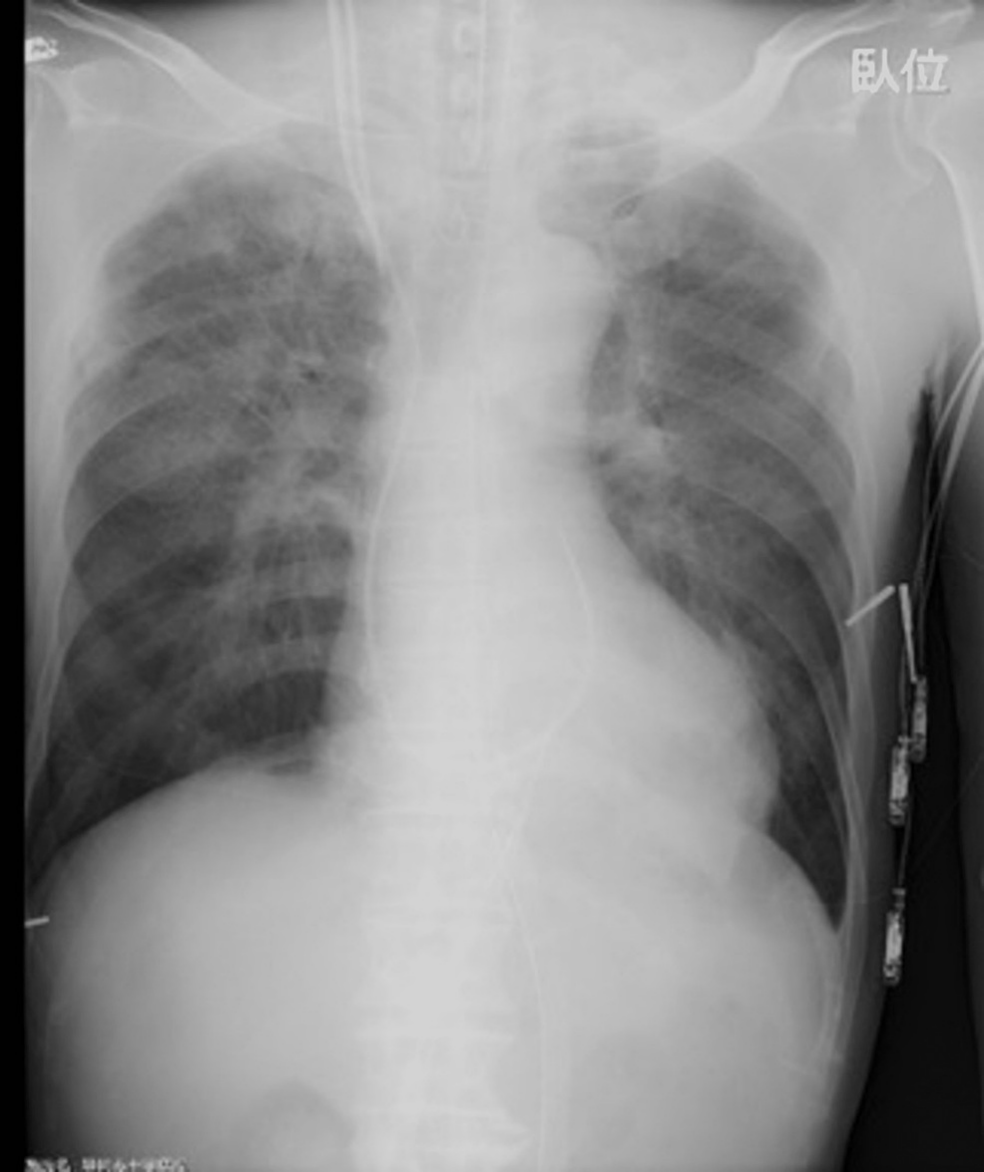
Postoperative chest radiograph. Bilateral pulmonary edema and disproportionately mild cardiomegaly with a cardiothoracic ratio of 53.5% were observed in the supine position.
